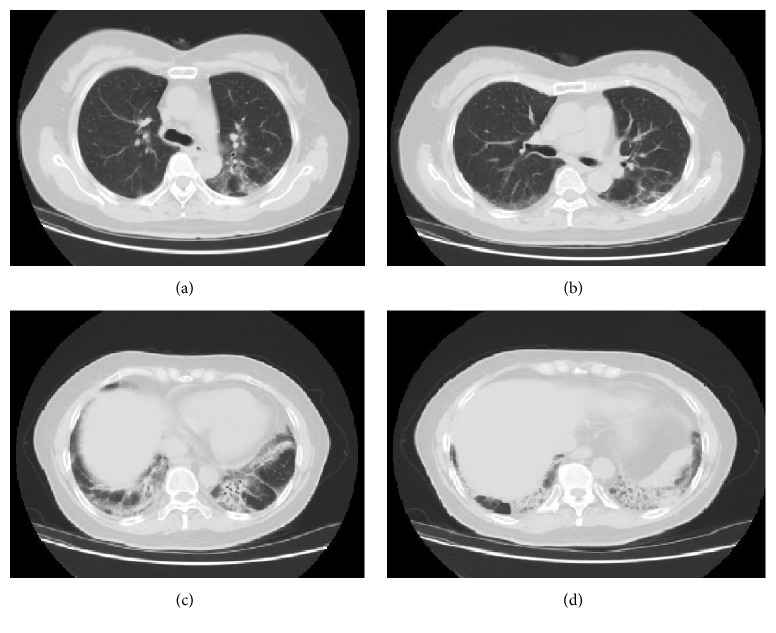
CT chest, axial views, showing bilateral peribronchial, lower lobe predominant ground glass opacities with evidence of early fibrosis as demonstrated by lower lobe volume loss and traction bronchiectasis.
